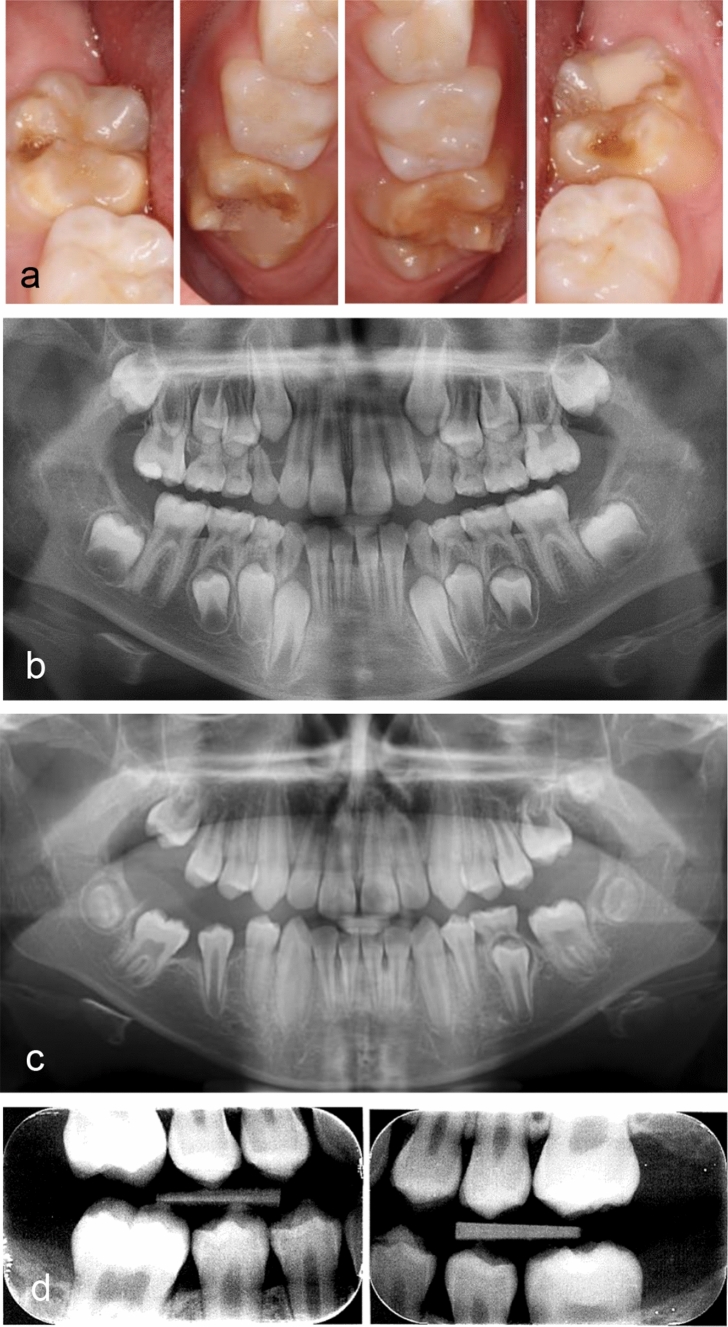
Good results after extraction of all FPM. a Clinical photographs before extraction at 8.5 years. Hypomineralised enamel are seen on all surfaces. Fillings have been placed after enamel breakdown in 16 and 36. The child experienced pain and hypersensitivity from all FPM and the child and parents preferred extractions instead of life-long restorative treatment need. b Panoramic radiograph before extraction at 8.5 years. c Panoramic radiograph at follow-up 1.7 years later when the child was 10.2 years. d Bitewing radiographs at 11 years show stable occlusion without spaces or tipping of second permanent molars
